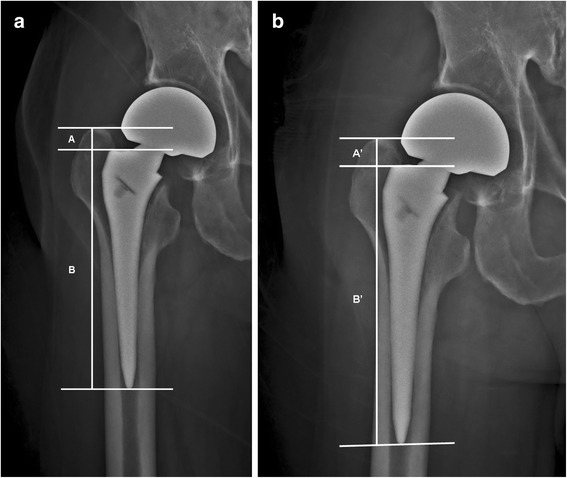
a Plain X-ray of the implanted femoral components taken immediately after surgery. The implant (PROFEMUR® Z) fitted at the metaphyseal-diaphyseal junction and the proximal diaphysis. The vertical distance between the lateral shoulder of the prosthesis and the superior tip of the greater trochanter on the radiograph was recorded as A; the distance from the lateral shoulder of the prosthesis to the distal tip of the prosthesis was recorded as B. b Plain X-ray of the implanted femoral components taken on follow-up. When measuring subsidence, corrections were made using the ratio of vertical distance between the lateral shoulder of the prosthesis to the distal tip of the prosthesis (B’/B). The corrected vertical distance between the lateral shoulder of the prosthesis and the superior tip of the greater trochanter was calculated using the equation: A X (B’/B). The subsidence was calculated as A’ – A X (B’/B)
